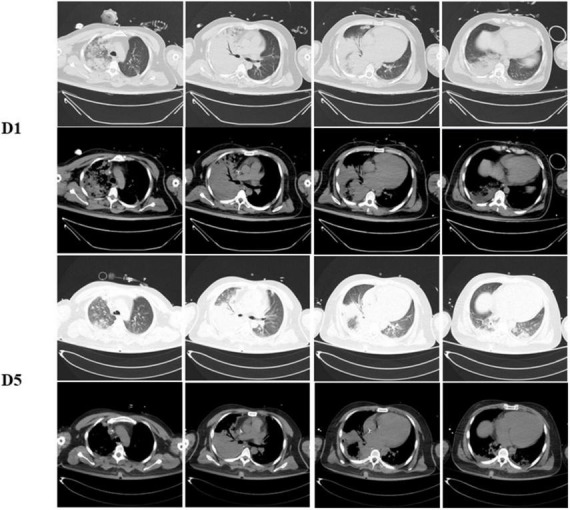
Dynamic change on computed tomography chest of the patient. Chest CT scans show with large area consolidation of the right lung on day 1 and improved after 5 days admitted.
